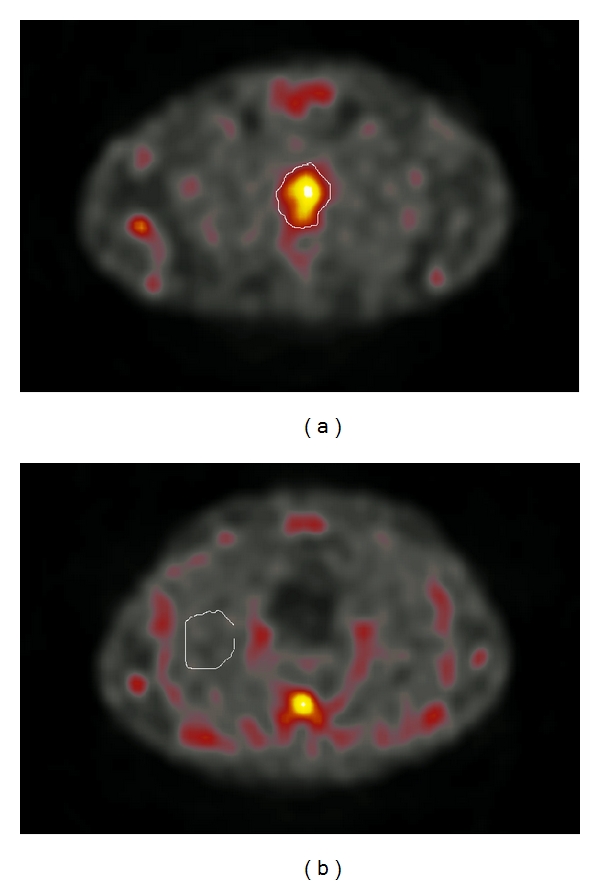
Evaluation of scintigraphic images with ^99m^Tc-depreotide. Region of interest (ROI) was drawn manually around the oesophageal tumour on each slice, using small margins, and a background ROI was drawn in healthy lung parenchyma.
